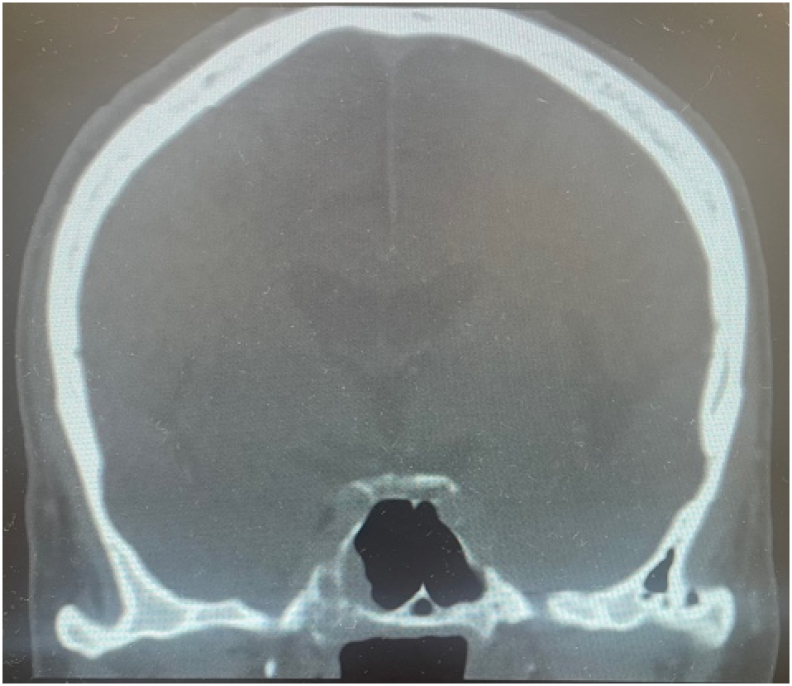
Coronal CT head showing 16 mm soft tissue lesion of right sphenoid with bony defects, extending into the cavernous sinus.
